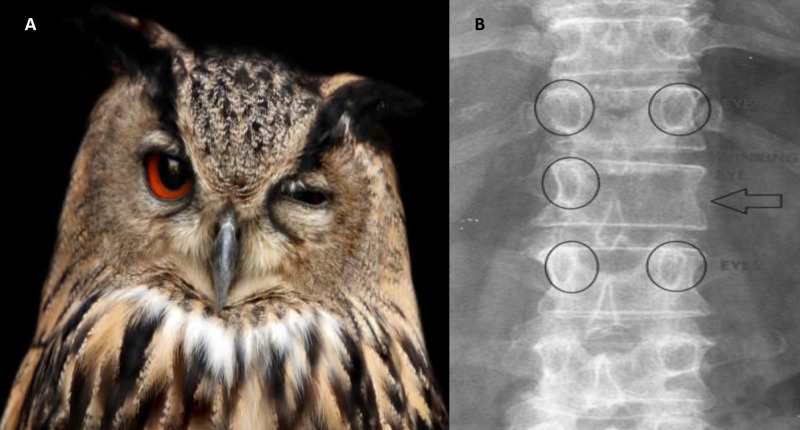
Winking owl sign A winking owl and x-ray showing similar appearance.
